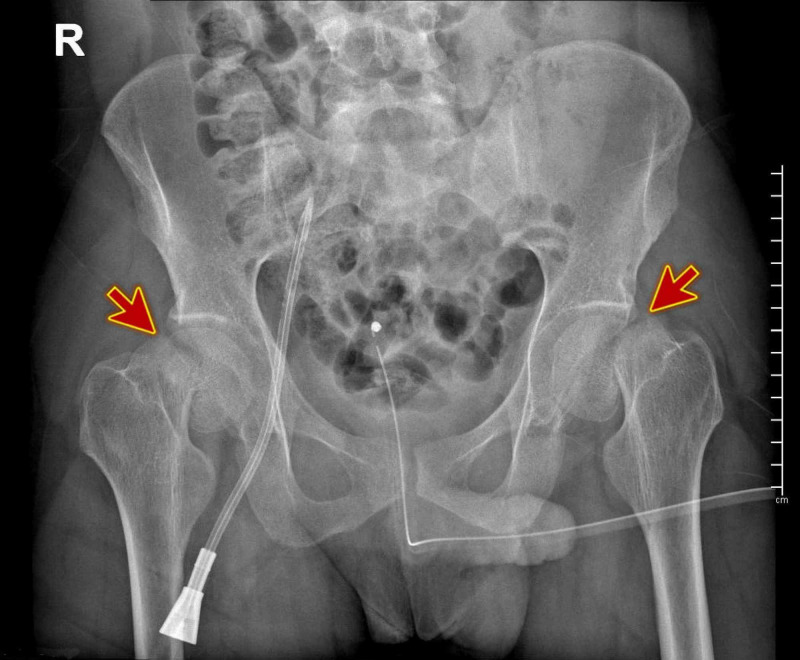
Pelvic X-ray showing bilateral displaced femoral neck fractures.
